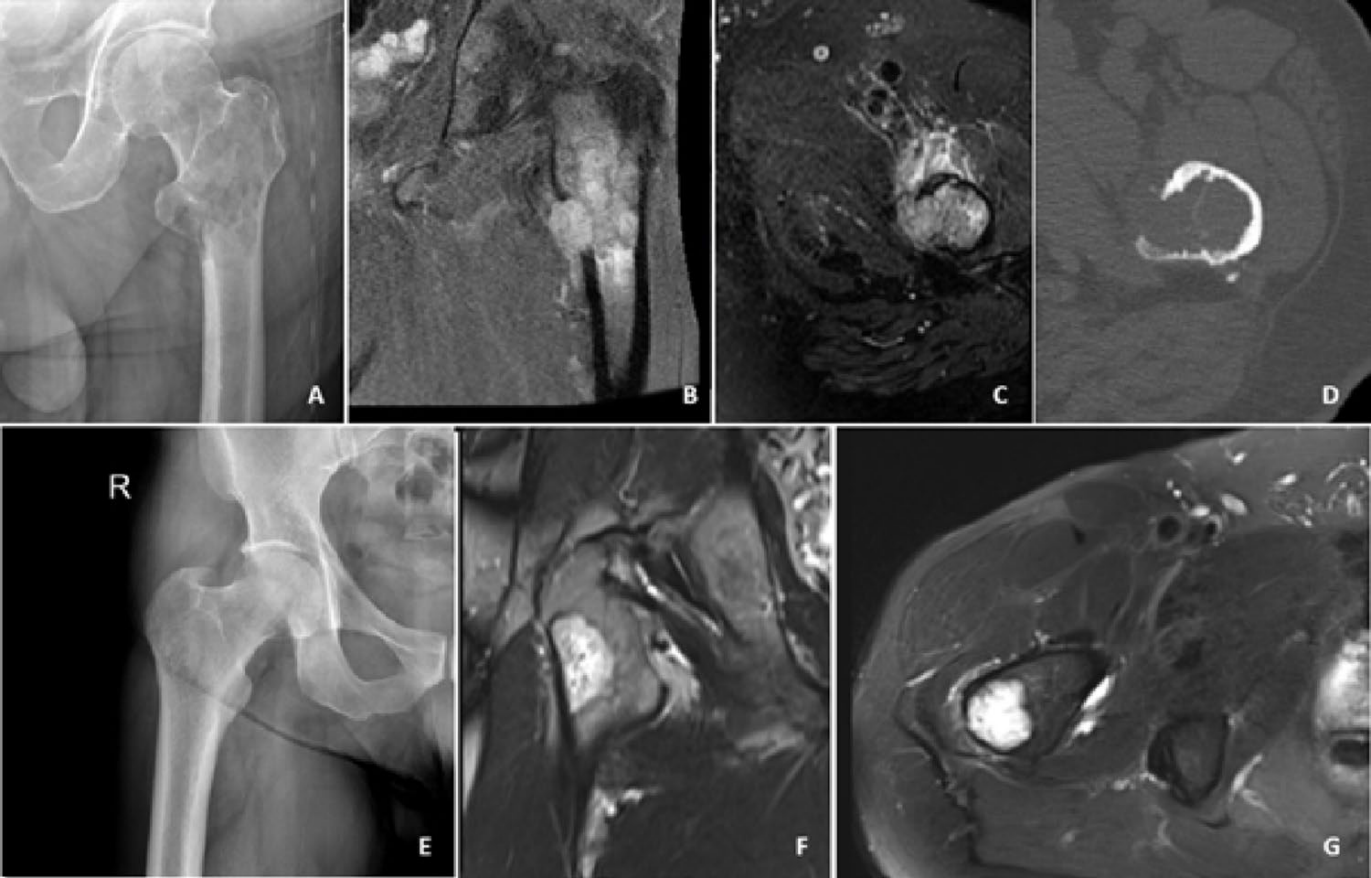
Benign vascular bone tumors may demonstrate aggressive radiological features overlapping with malignant lesions. A–D) 72-year-old male patient with subtrochanteric epithelioid hemangioma; radiographs, MRI, and CT slices show a pathological fracture and a soft tissue component. E–G) 54-year-old male patient with epithelioid hemangioendothelioma; radiographs and MRI slices show an eccentric medullary lesion without cortical destruction or expansion
